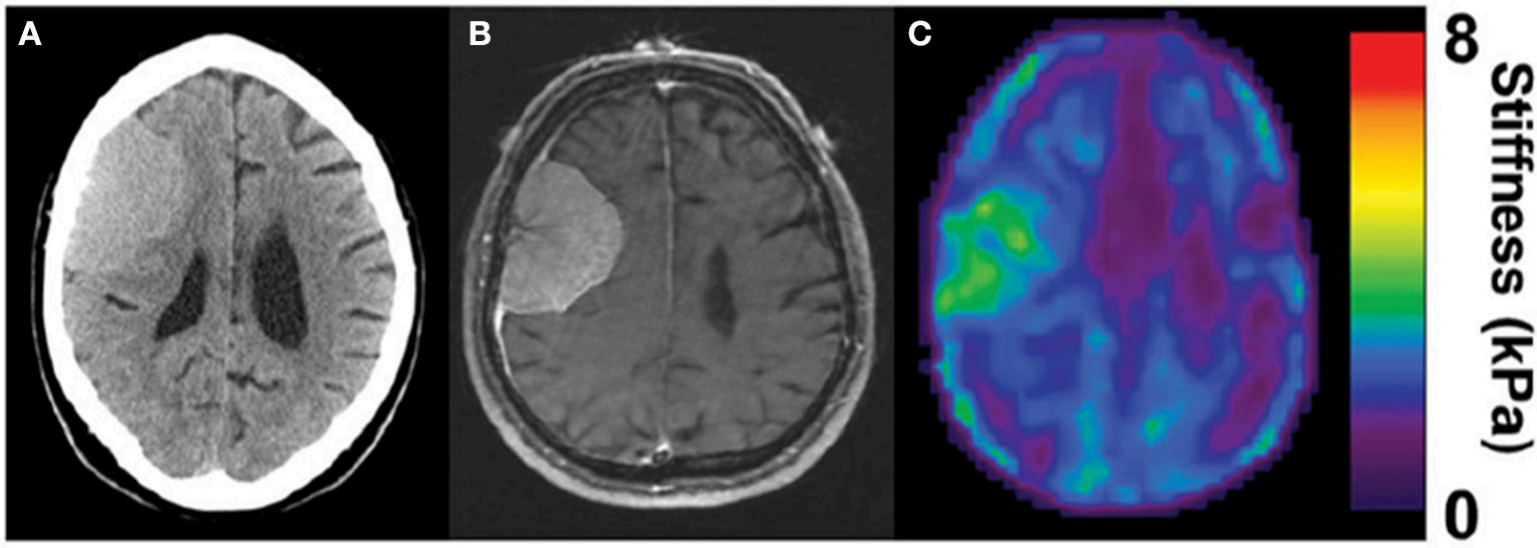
Stiffening of meningioma as measured by MRE. Adapted from Hughes et al. (2015) by permission of Oxford University Press. (A) CT image of the head, (B) corresponding MRI, and (C) corresponding MRE image showing homogenous tumor with the stiffness greater than surrounding healthy tissue (in green).
